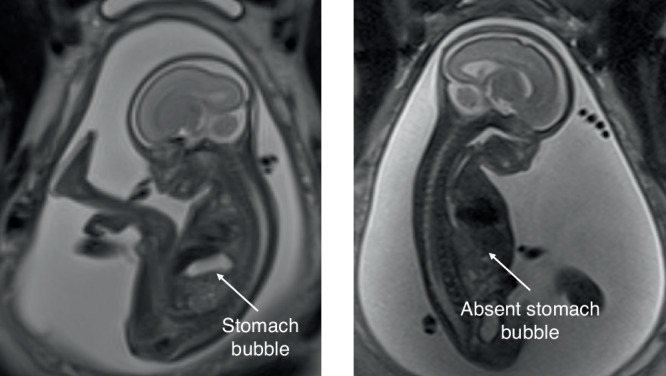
Comparison of normal and absent stomach bubble. Sagittal T2 SSFSE fetal MRI images of normal stomach bubble size in a 22-week fetus (left) in comparison with an absent stomach bubble in a 30-week fetus with TOF/OA (right).
